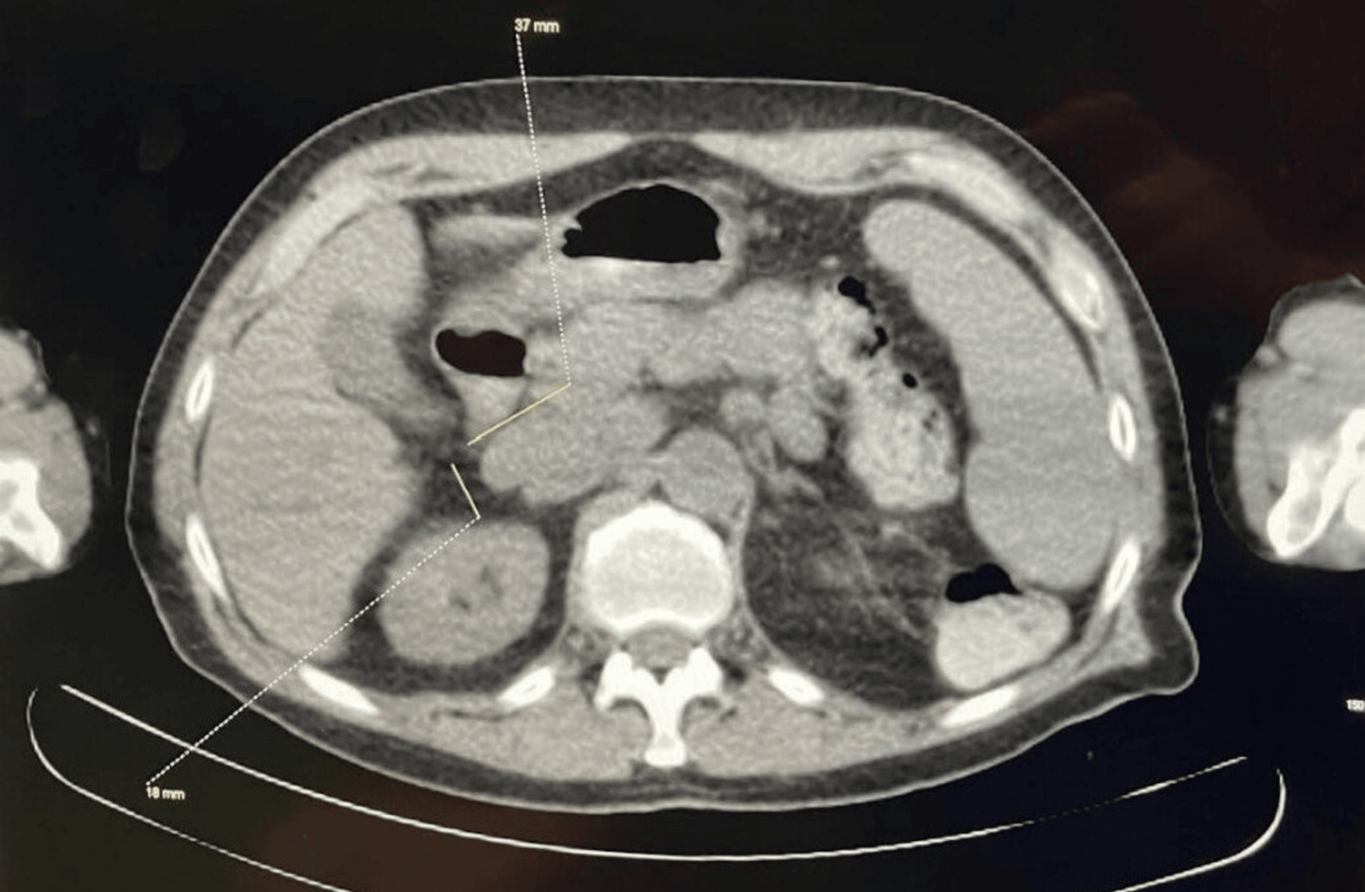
PET-CT scan showing low-grade metabolic activity in an enlarged anterior portacaval node.
